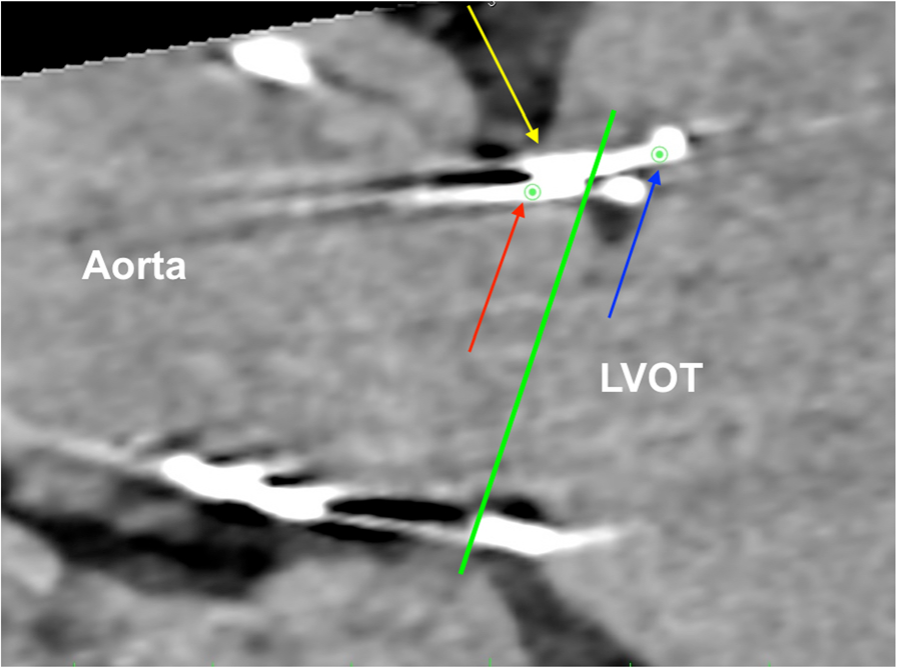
Additional planning of intervention using cardiac computed tomography: given is the analysis for left coronary cusp (analyses of right and non-coronary cusp are not shown). *Red arrow*, “feeler” of JenaValve; *blue arrow*, lowest point of JenaValve; *yellow arrow*, aortic annulus in at deepest point in left coronary cusp; *green line*, intended level for positioning of the lowest point of CoreValve Evolut R prosthesis determined by our Transcatheter aortic valve implantation-Team (not above the original aortic annulus and not deeper than lowest point of JenaValve avoiding possible interference with mitral valve). *LVOT* left ventricular outflow tract
